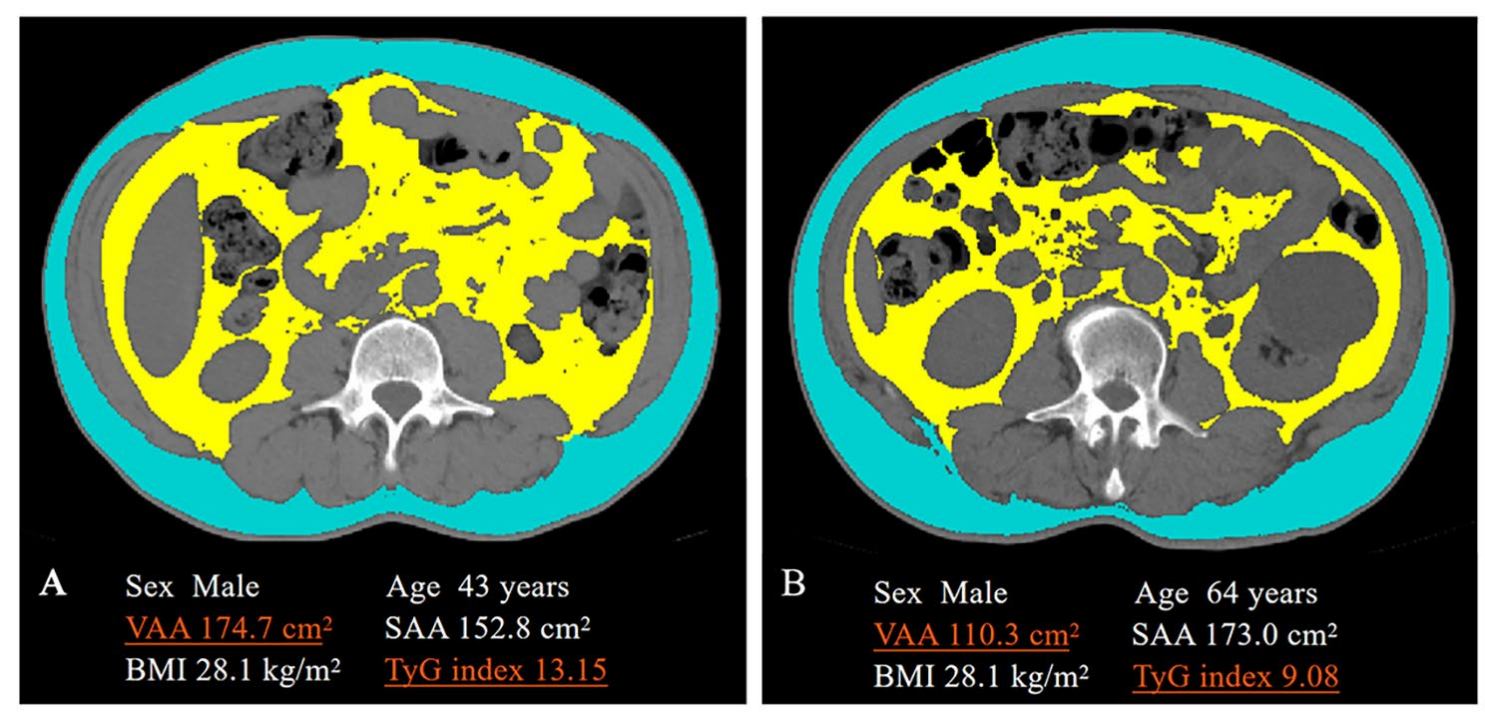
CT image analysis at the lumbar 3 level using Slice-O-Matic software. Blue: subcutaneous adipose tissue; yellow: visceral adipose tissue; grey: unprocessed CT image
